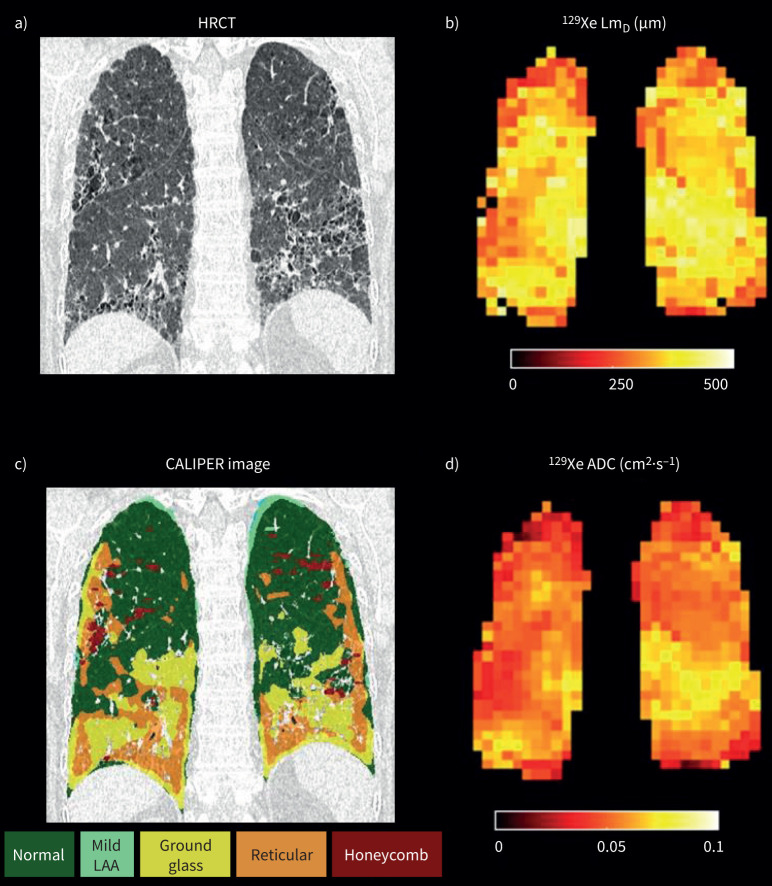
a) High-resolution computed tomography (HRCT) image, b) 129-xenon (^129^Xe) mean acinar dimension (Lm_D_) map, c) Computer-Aided Lung Informatics for Pathology Evaluation and Rating (CALIPER) image and d) ^129^Xe apparent diffusion coefficient (ADC) map in an idiopathic pulmonary fibrosis subject. LAA: low-attenuation area.
